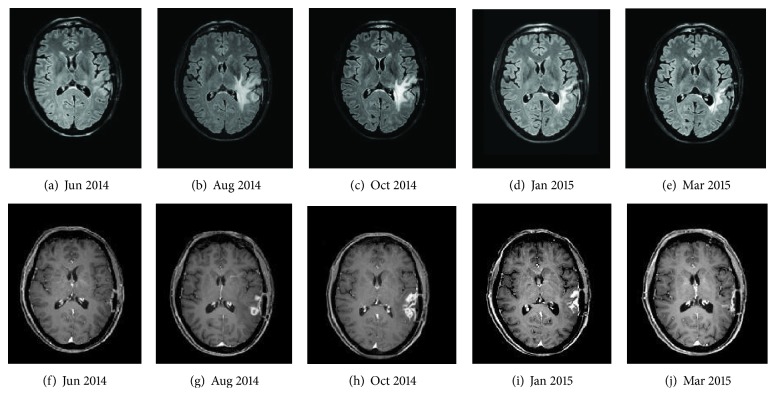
FLAIR (a–e) and contrast-enhanced T1-weighted images (f–j): postsurgical (a, f), increasing edema (b, c), enhancement (g, h) and subsequent reduction (d, e, i) of both, and remission of the enhancing lesion (j) in the course of immunotherapy with dendritic cell vaccine.
